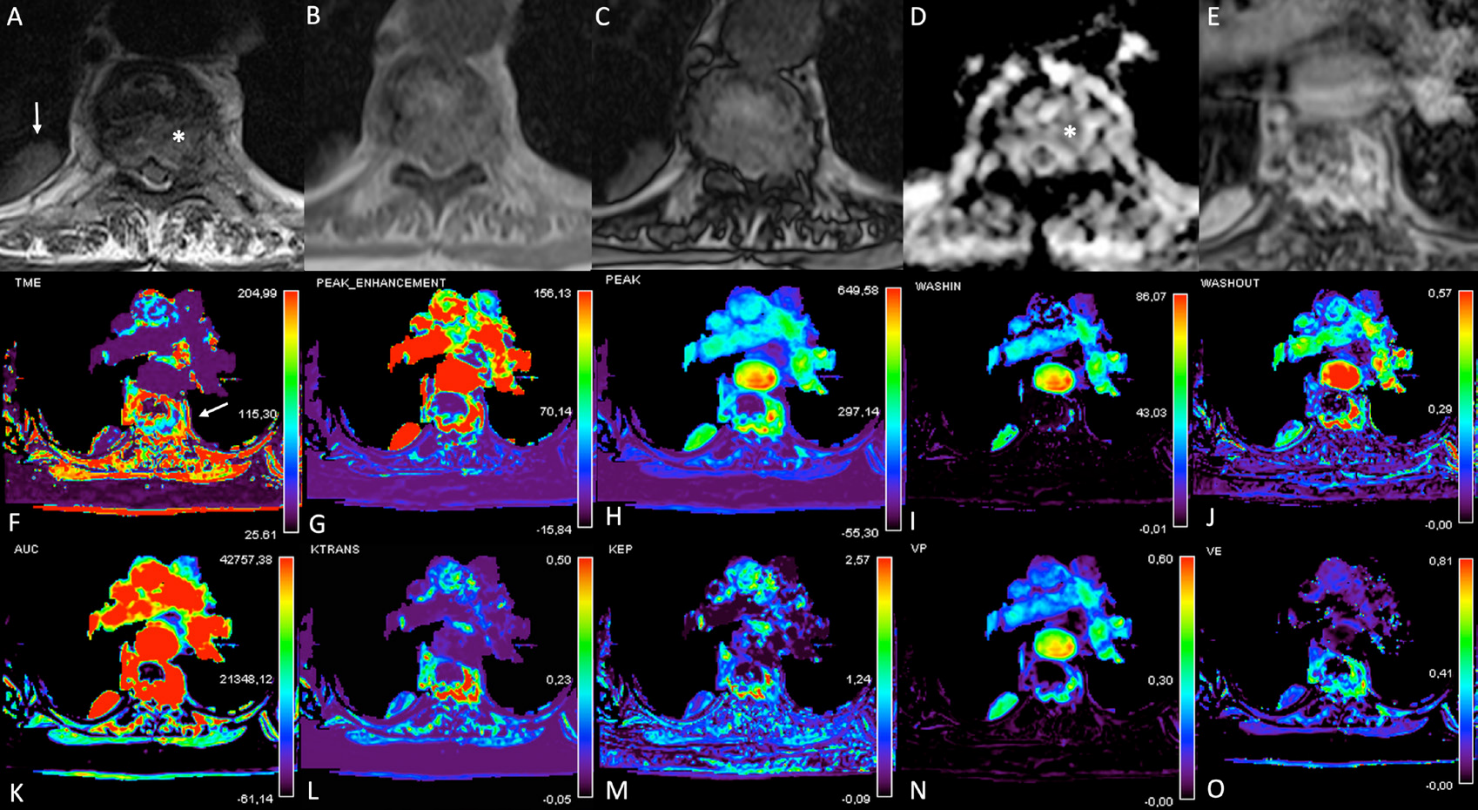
MRI multiparametric evaluation of a malignant VCF. Axial *T*
